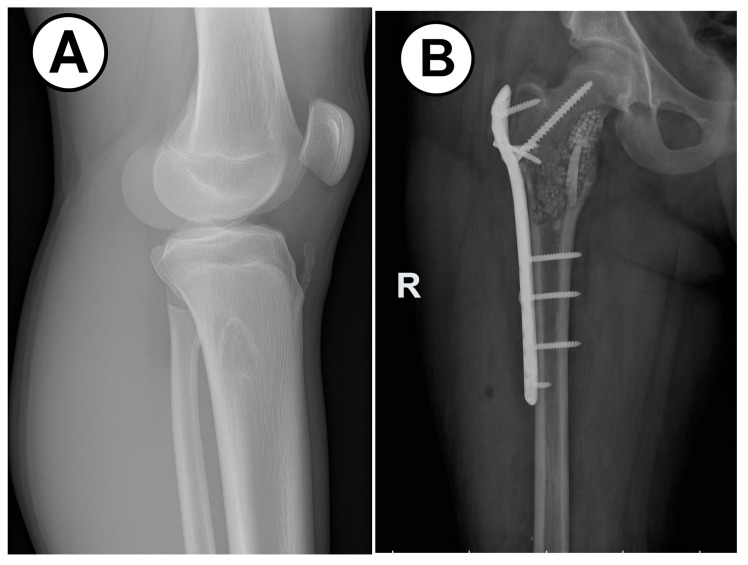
Fibrous dysplasia, imaging aspects. (A). Lateral X-ray of fibrous dysplasia of the left tibia. The lesion appears to have well defines sclerotic borders and is located at the meta-diaphyseal region. (B). Treated fibrous dysplasia. Postoperative antero-posterior view of the left femur with bridging osteosynthesis using anatomic plate and screws, femoral fenestration, enucleation of the tumoral mass, and packing with a bone substitute. (The cases refer to distinct patients.)
